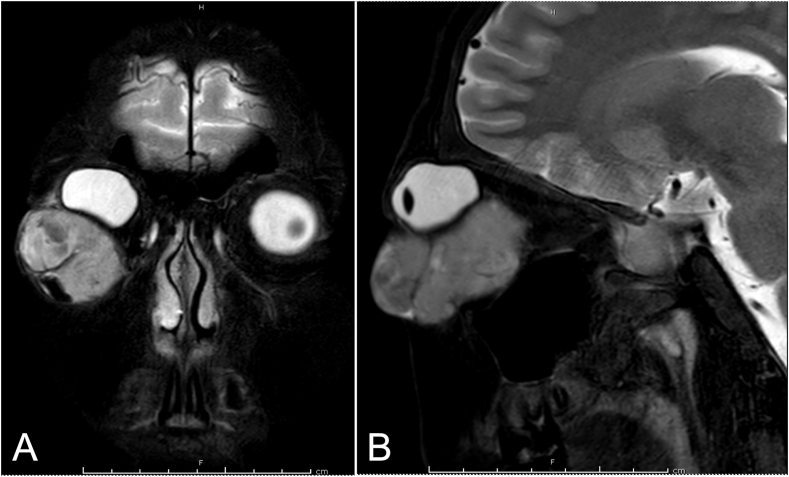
Orbital T2-weighted MRI with fat suppression of the patient at 3 months after the first presentation. (A) Coronal view. (B) Sagittal view.
